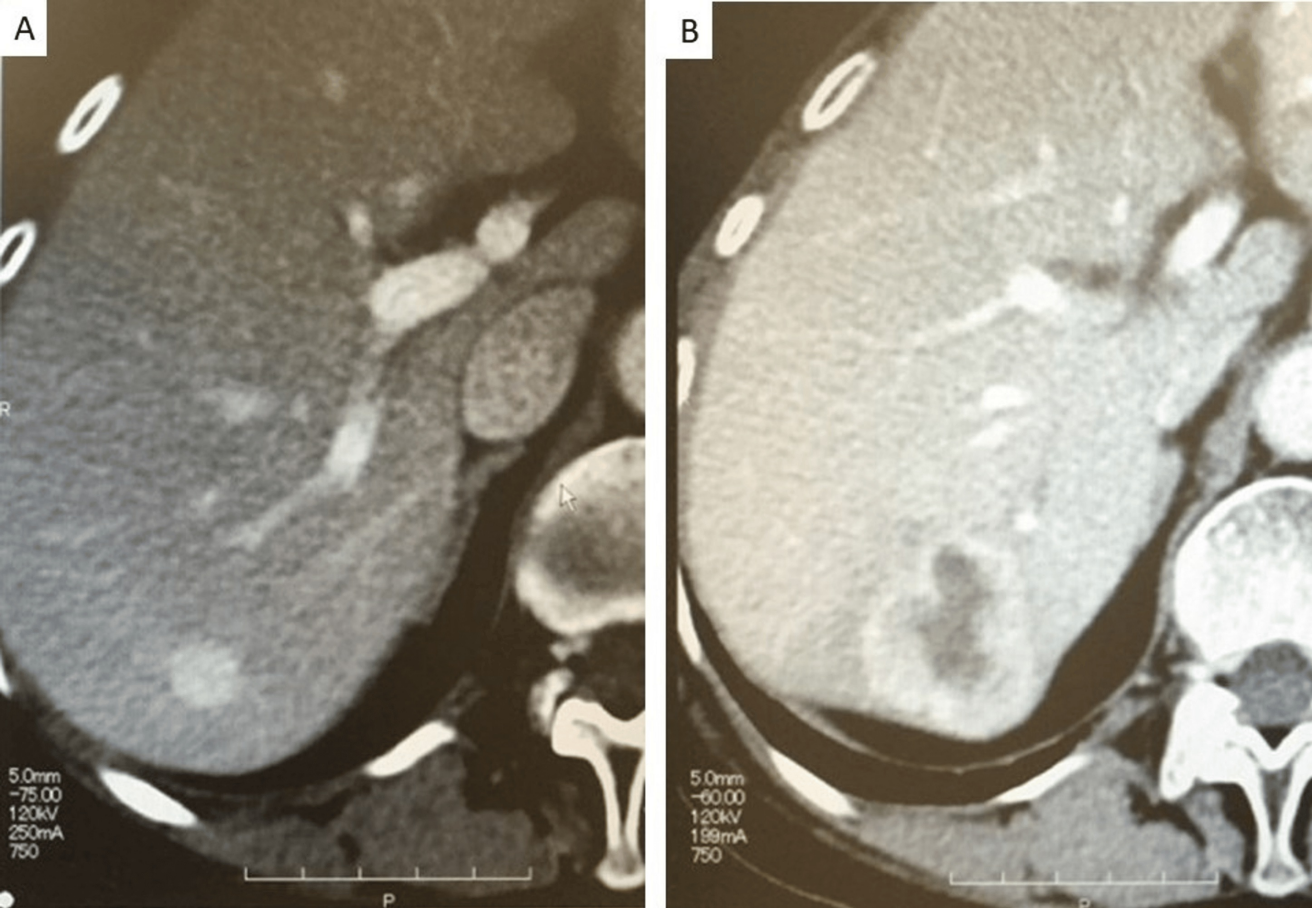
Contrast-enhanced CT images; (A) seven years prior, and (B) current presentation. A: A 12-mm tumor with uniform contrast enhancement is seen in the liver. B: The tumor diameter has increased to 35 mm, with irregular margins and minimal internal contrast, suggesting vascular attenuation.
